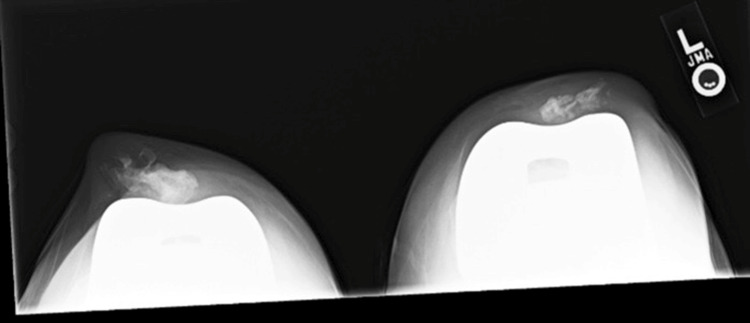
Preoperative sunrise radiograph of bilateral knees demonstrating resorption of both patellae and subluxation of the patellar implant components
